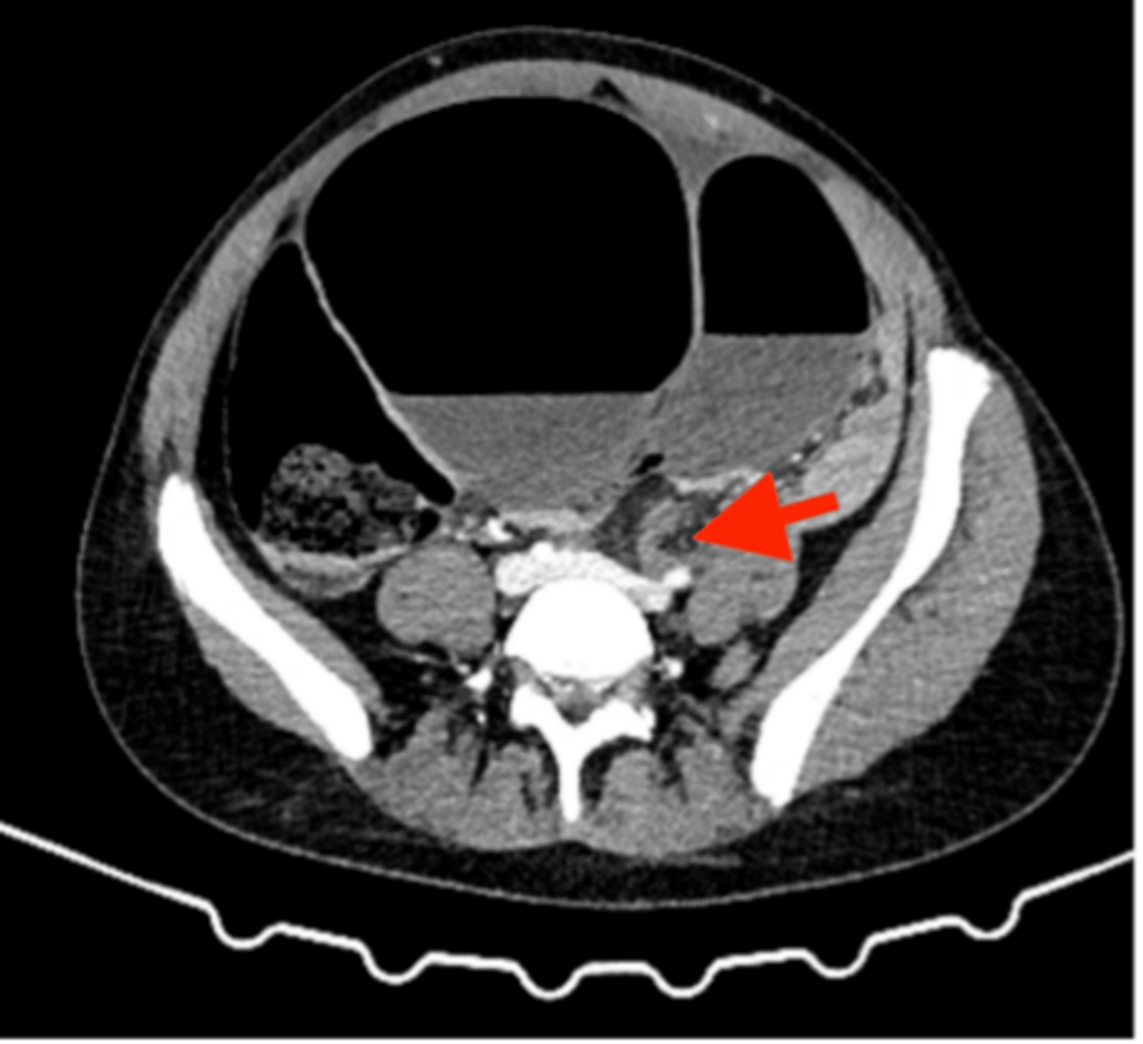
Abdominal computed tomography (CT) showing the swirling sign and transition point indicated by the red arrow
